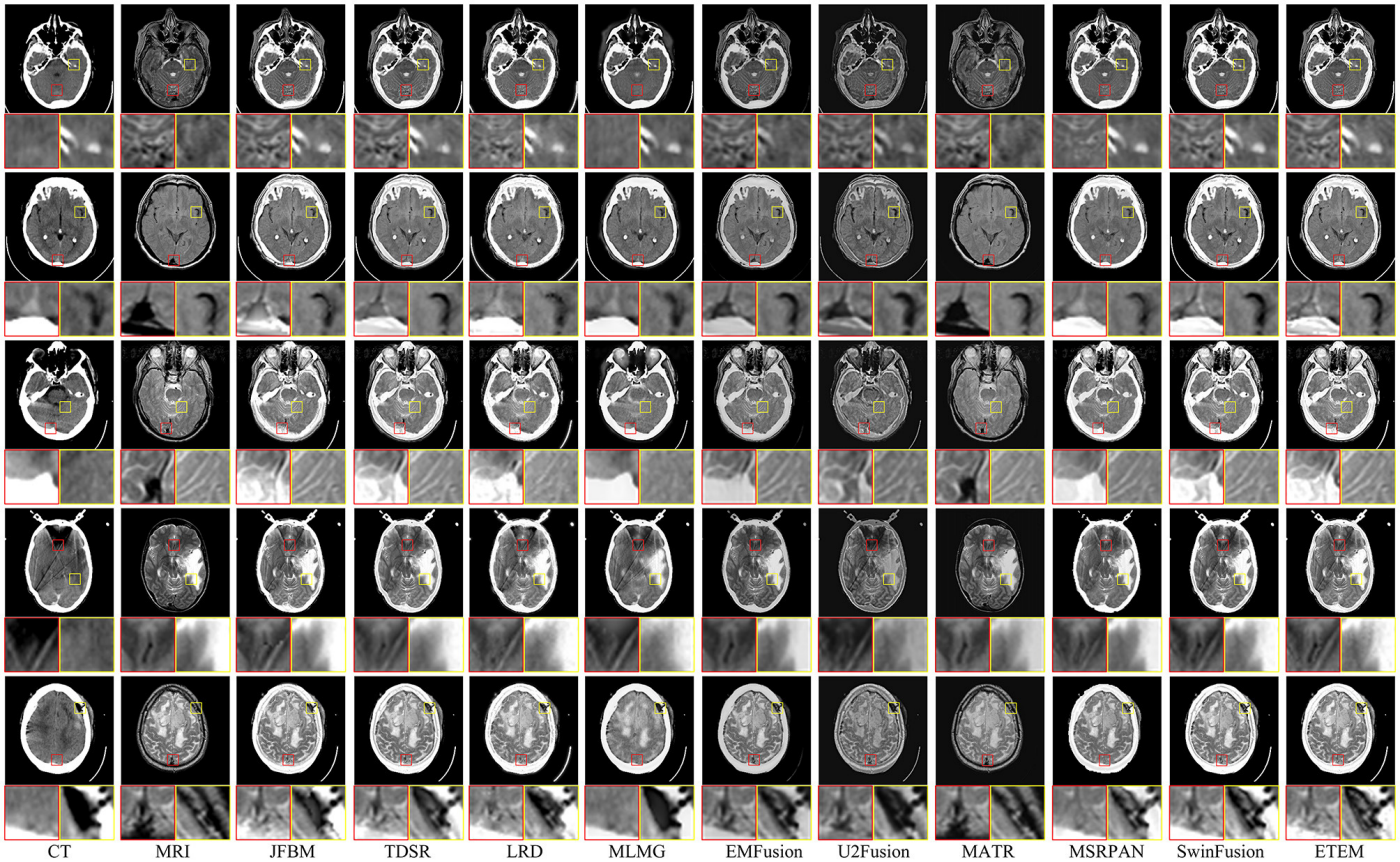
Qualitative comparison of different methods on five CT and MRI image pairs.
